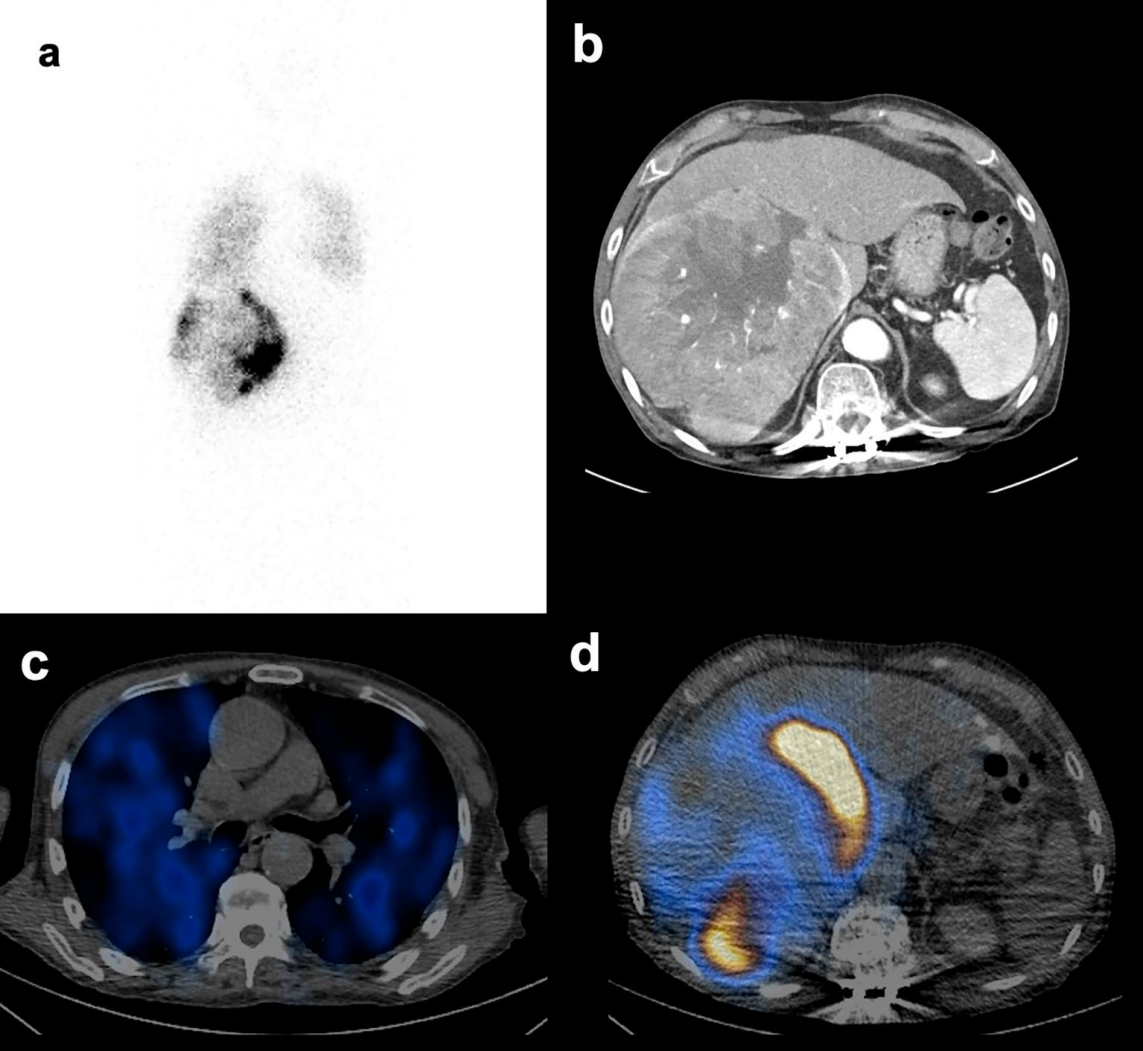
Representative case of a patient with high LSF. The patient (76-year-old male) had an LSF of 29.1% on the planar scan (a). He had a large sized tumor (1730 L) with central necrosis, and an integral total (HU × L) value of 141.28 (b). From the SPECT/CT, LSF was 14.1% (c), and the tumor uptake was 82.1% (d).
